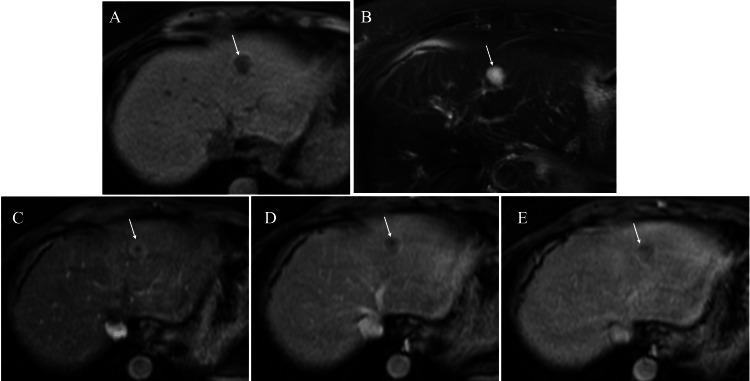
Magnetic resonance imaging (MRI) findings (A) Fat-suppressed T1-weighted images of the tumor showed low signals (arrow). (B) Fat-suppressed T2-weighted images of the tumor showed high signals (arrow). (C) Contrast images of the tumor showed rim enhancement (arrow) on arterial phase images. (D) Contrast images of the tumor showed very weak enhancement (arrow) on portal phase images. (E) Contrast images of the tumor showed retained very weak enhancement (arrow) on late-phase images.
